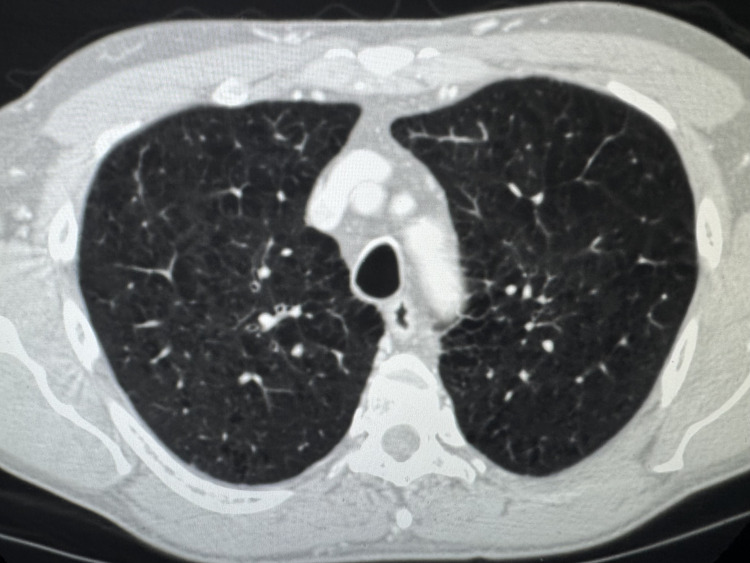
Axial section of low-dose chest CT showing bilateral, upper, and middle lung centrilobular emphysema CT: computed tomography
